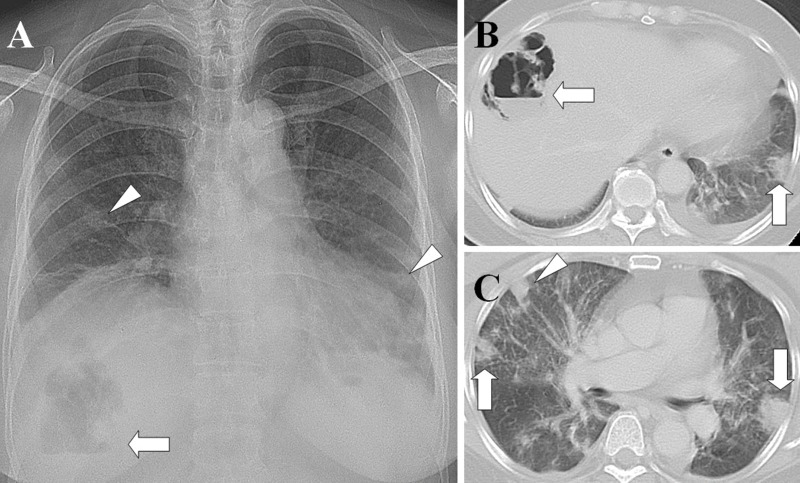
Liver abscess with septic pulmonary emboli. (A) A chest radiograph shows an air-fluid level in the right upper abdomen (arrow) and multiple nodular opacities in the bilateral lungs (arrowheads). (B) A CT scan shows a gas-forming liver abscess (arrow) and a peripheral wedge-shaped opacity abutting the adjacent pleura (arrow). (C) A lung window of a cross-sectional CT scan shows two peripheral wedge-shaped opacities abutting the adjacent pleura (arrows) and a peripheral nodule with a feeding vessel (arrowhead). The patient was a 61-year-old diabetic woman whose blood and aspirate abscess cultures were positive for *Klebsiella pneumoniae*.
